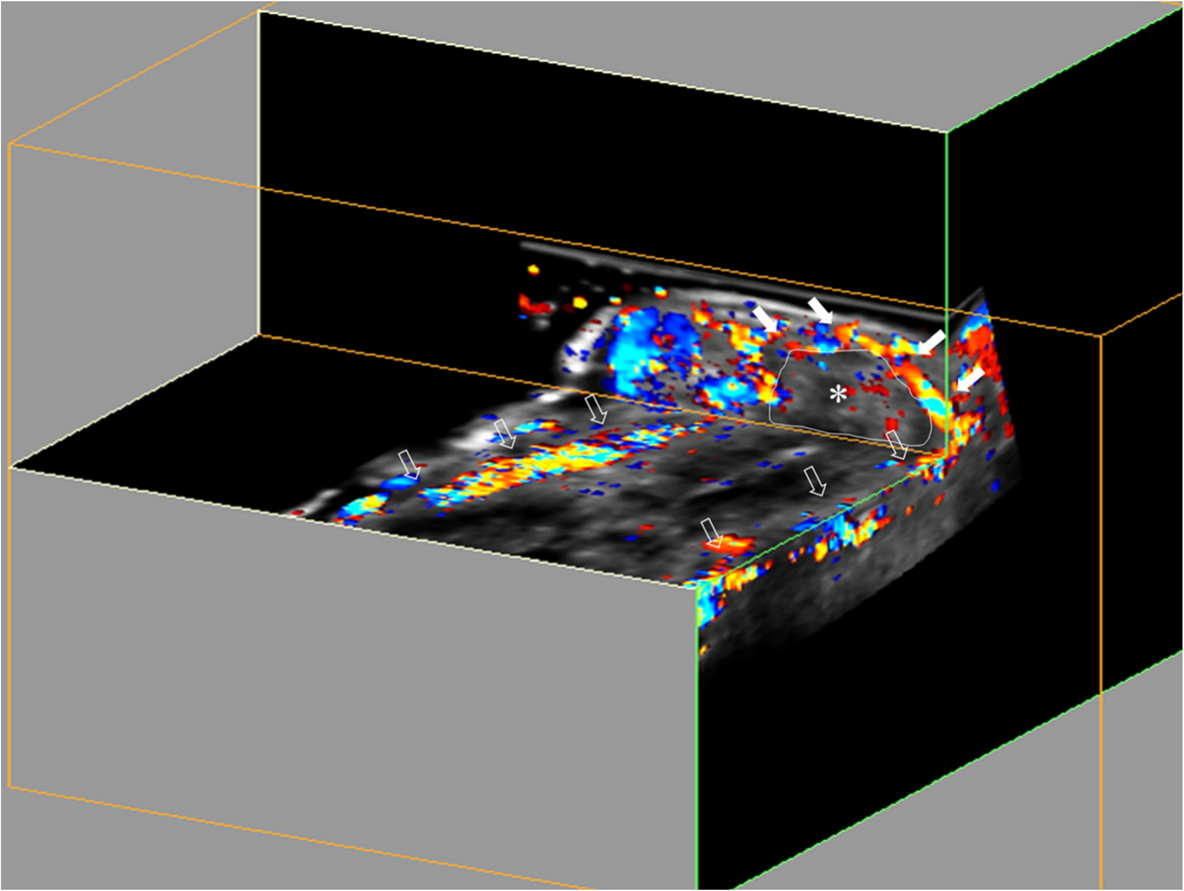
An ultrasound volume video clip of the palmar interphalangeal part of the second finger demonstrates two digital arteries (*open arrows*) and a transverse feeding vessel (*white arrows*). *Flexor tendon and sheath of the second finger. Due to the close proximity to the tendon sheath of the feeding vessel, blooming or reverberation may be misinterpreted, because they seem to be located inside the tendon sheath
